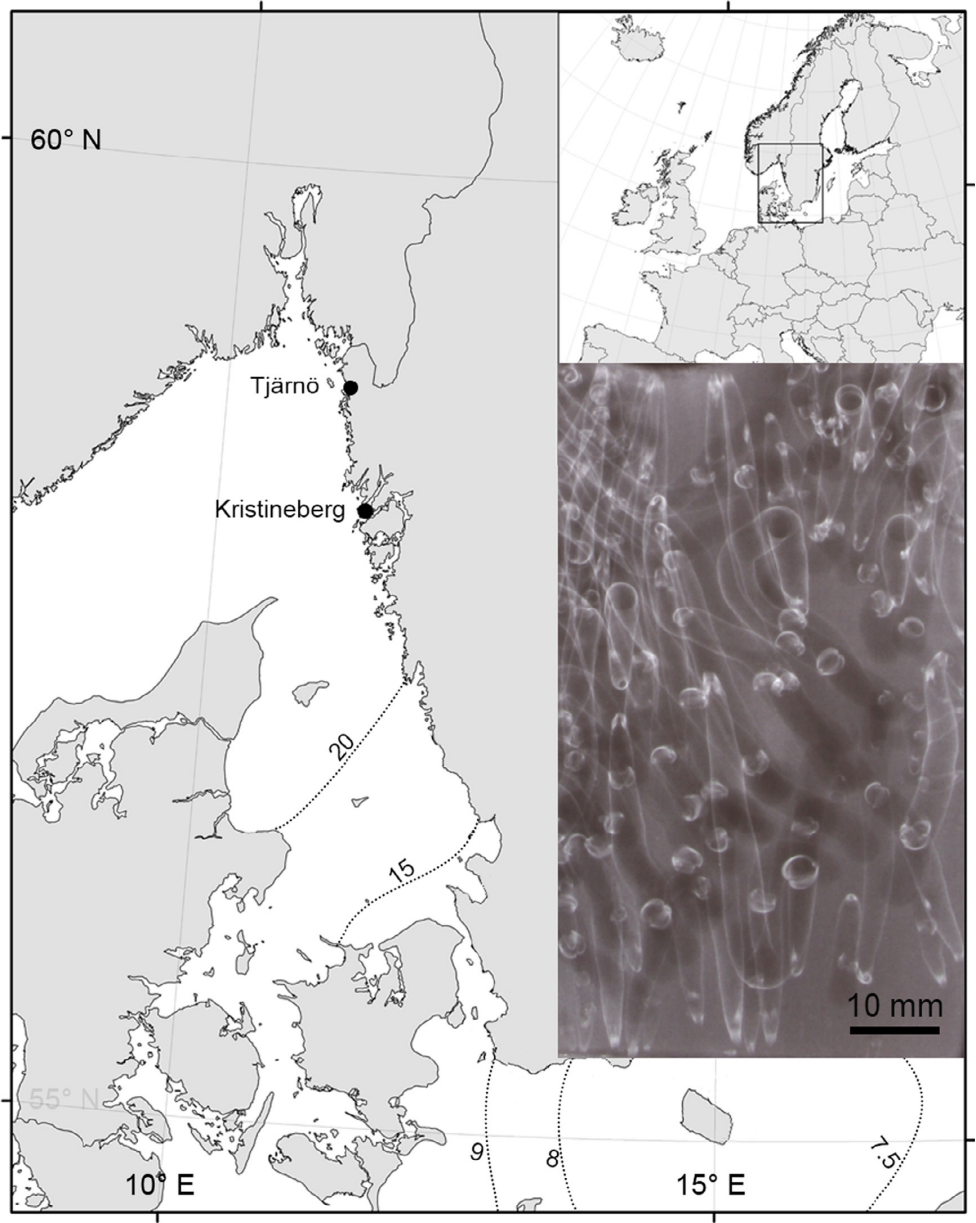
Location of the study sites. Dotted lines indicate isohalines, numbers indicate mean annual surface salinity. Inset: radiograph of part of a shipworm‐infested panel.
